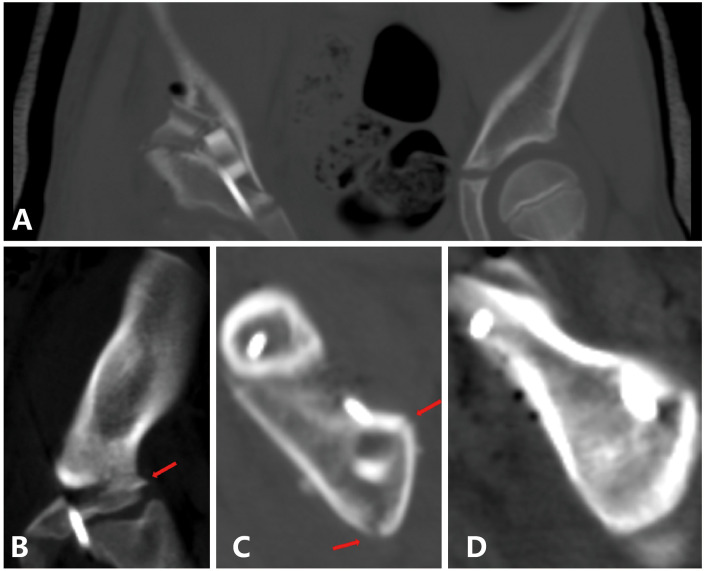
Distraction and fixation following an MPO osteotomy. (A) Postoperative CT scan of right DDH showing outward rotation of the osteotomy fragment. (B) Sagittal CT View. The red arrow indicates a greenstick fracture at the sciatic notch. The Y-shaped cartilage and superior bone structures remain intact. (C) Axial CT View at Osteotomy Level. The inferior arrow marks the terminus of the external iliac plate osteotomy, which serves as the center of rotation, with outward rotation of the distal segment. The superior red arrow indicates opening of the internal iliac plate. The femoral autograft is fixed within this gap, positioned closer to the sciatic notch compared to the conventional periacetabular osteotomy. (D) Axial CT View Below Osteotomy Level. At this adjacent inferior level, the iliac cortex is continuous, and the osteotomy line is no longer visible.
